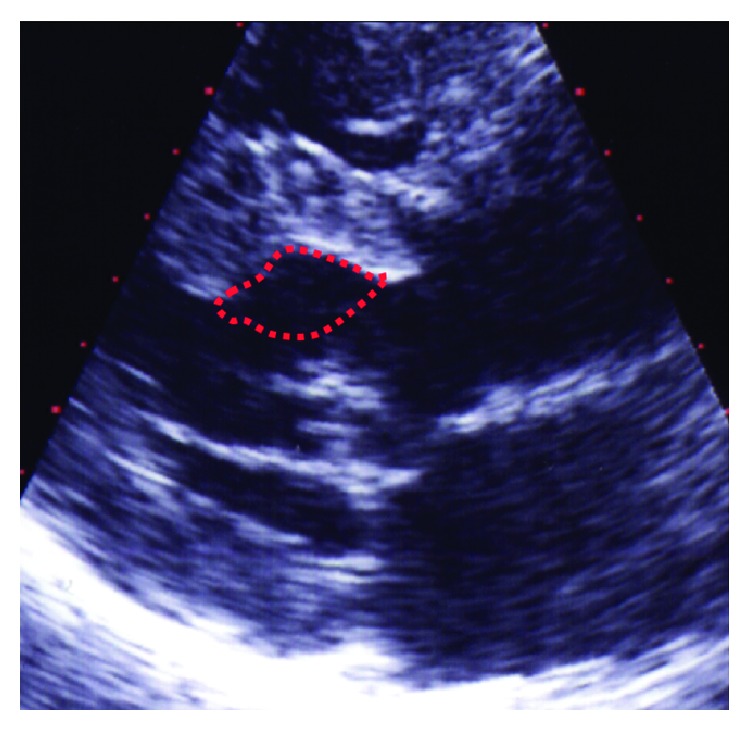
Echocardiographic visualization of the septal trough (dotted line) produced by a myectomy procedure.
